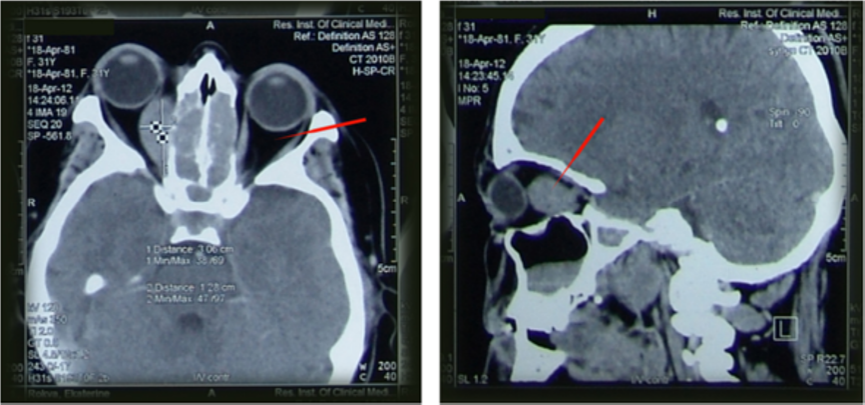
A computed tomography scan of the paranasal sinuses shows heterogeneous structural opacification of the ethmoidal, sphenoidal and frontal sinuses, mainly at the soft tissue; low-density opacification of the maxillary sinuses, mainly of the viscous fluid; and oval-shaped soft neoplastic tissue mass, 3.1 × 1.3 mm with sharp margins on the medial rectus of the right orbit that caused anteriotemporal dislocation of the eyeball (red arrow).
